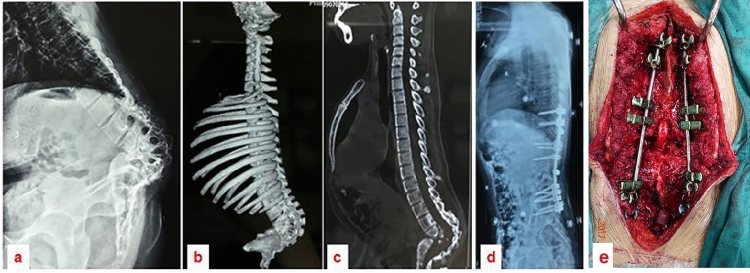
27-year-old female patient with severe sharp angular kyphosis due to Pott’s disease. Image (a) shows a lateral radiograph of the entire spine. Images (b and c) display 3D CT scans of the spine, highlighting the sharp angular kyphosis affecting the lumbosacral region. Image (d) is a postoperative lateral radiograph in which satisfactory correction is evident subsequent to an osteotomy at T12. Image (e) exhibits an intraoperative view of the spinal cord at T12 following a three-column osteotomy.
